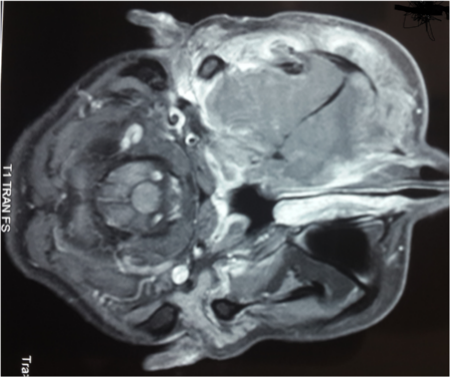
Axial T1-weighted magnetic resonance image of the facial area showing an invasive tumor occupying the right infratemporal fossa and maxillary sinus with irregular boundaries, heterogeneous intermediary signal at T1 weight, with sphenoidal, ethmoidonasal, and nasopharyngeal extension
